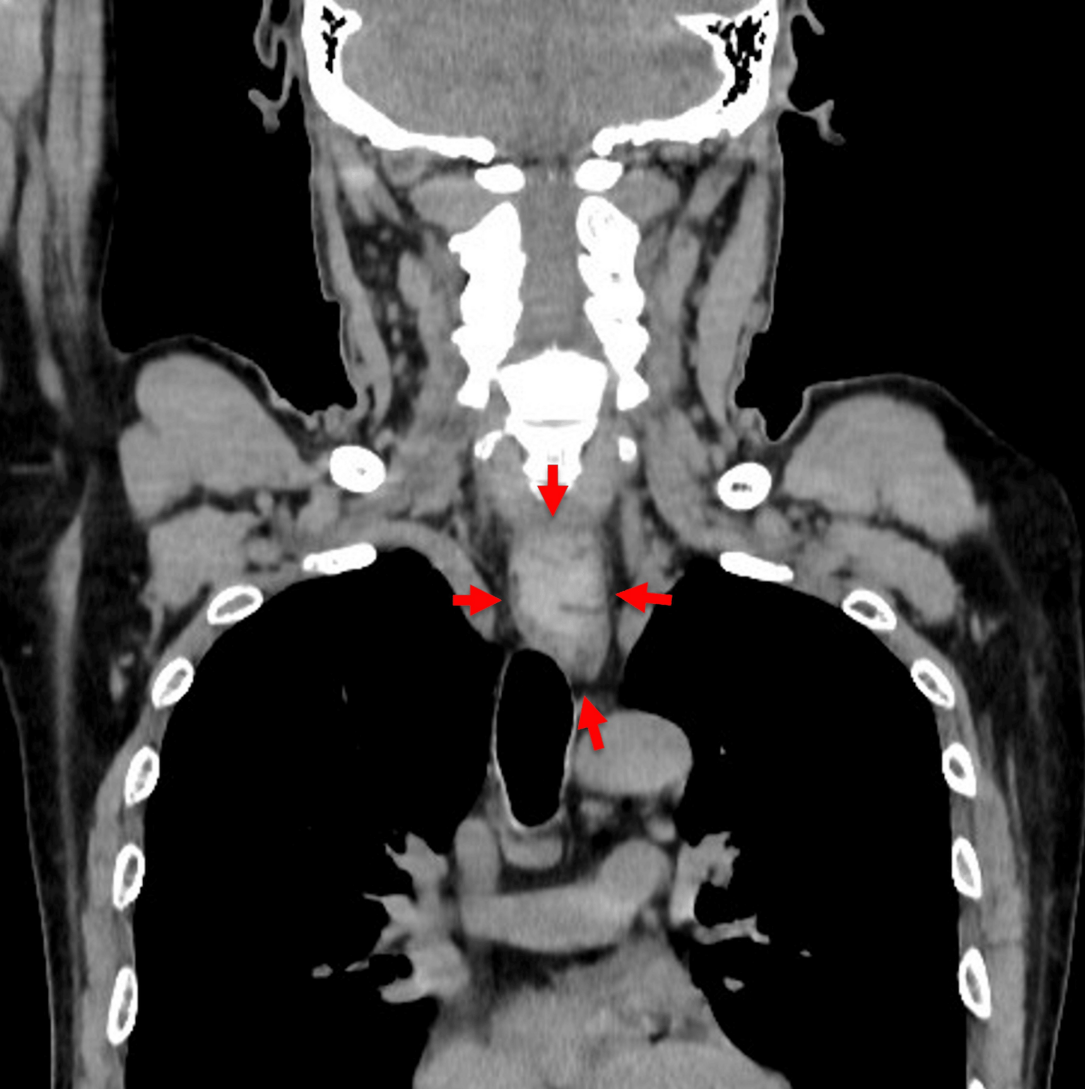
Coronal contrast-enhanced computed tomography (CT) image Coronal contrast-enhanced CT image of the chest showed a hyperdense object (arrows) lodged in the thoracic esophagus.
